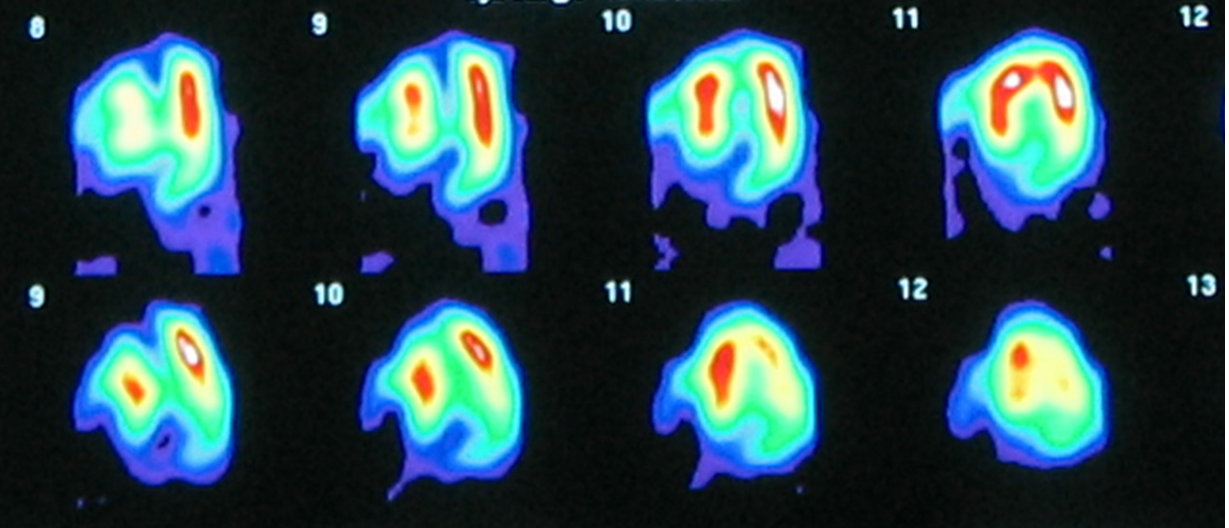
Gated-SPECT horizontal long axis end-diastolic image at rest showing a severe apical perfusion defect.
